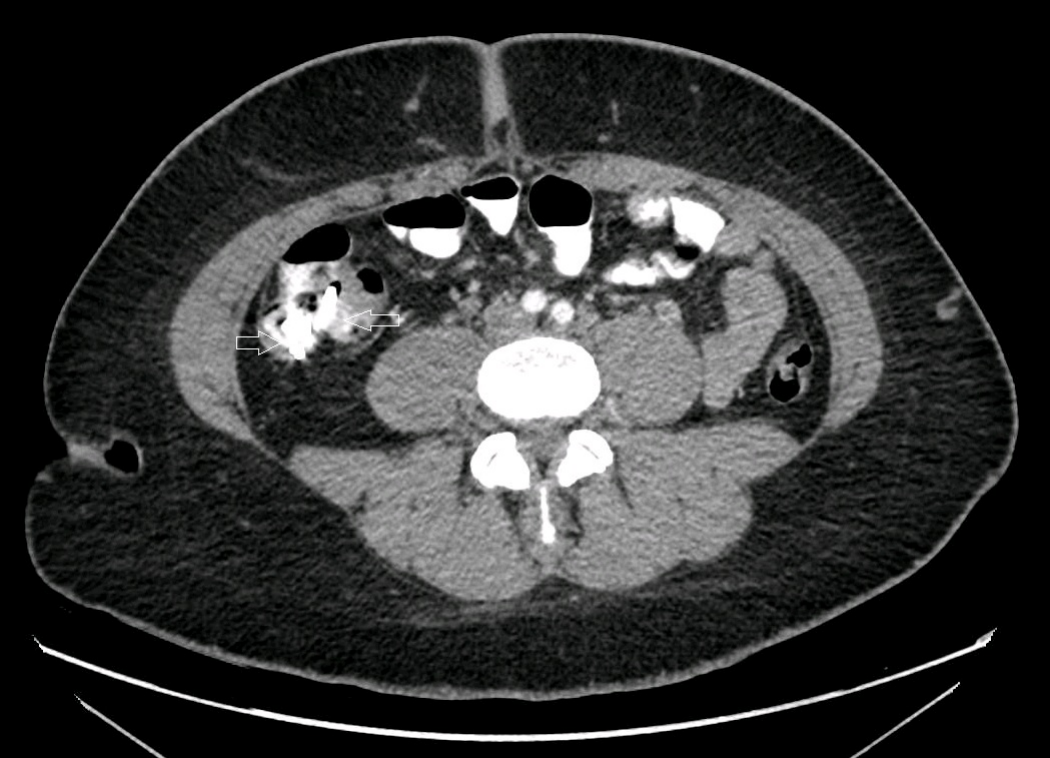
Abdominal computed tomography scan Hyperdense discs at arrow tips, located in the cecum.
